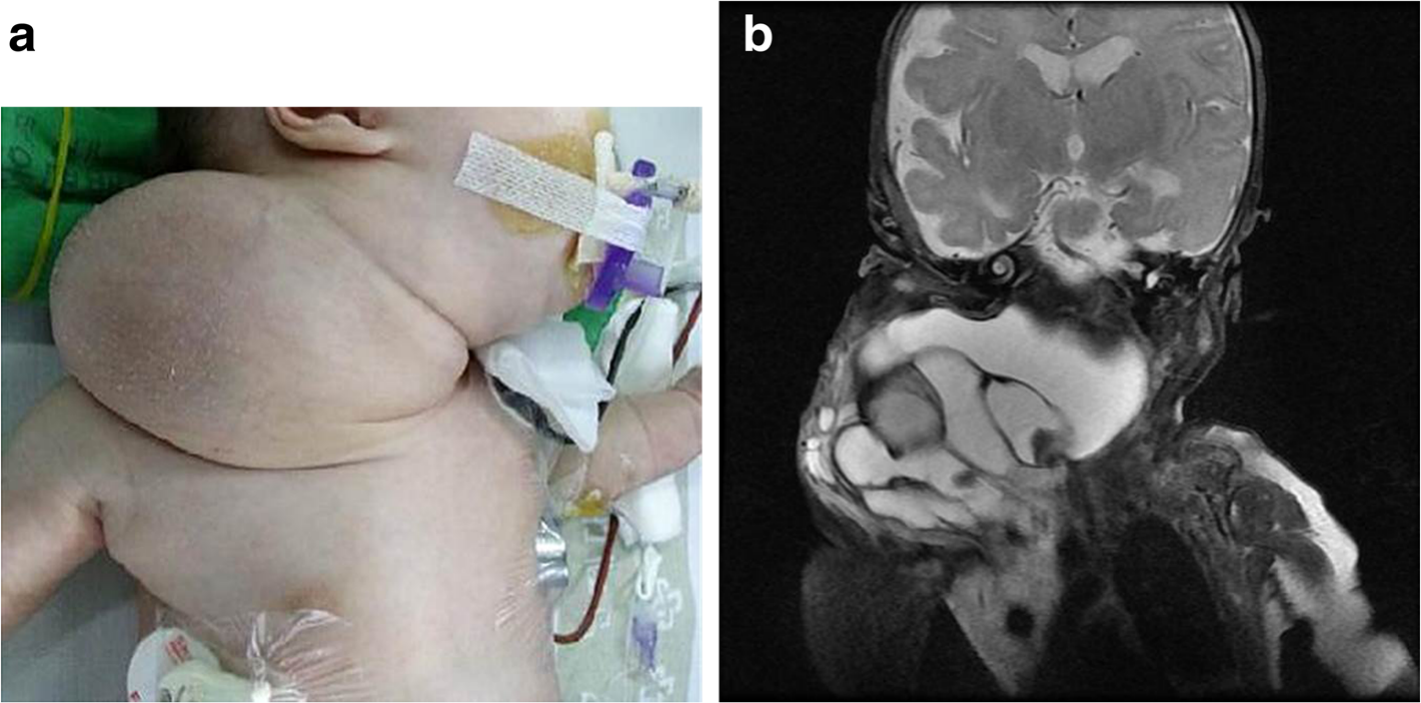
a Case 1. Photograph of a 41-day-old infant with a large cervical lymphatic malformation. b Case 1. Large cervico-mediastinal lymphatic malformations that encased almost all of the cervical vital structures, causing airway obstruction, visualized on magnetic resonance imaging
